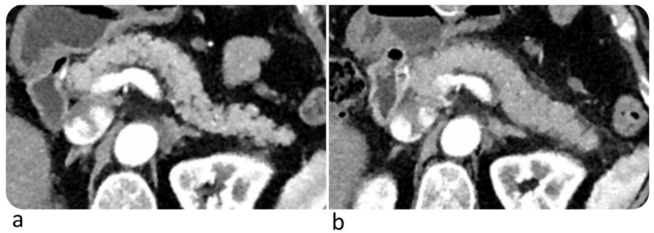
Complicated immune-related pancreatitis in a 61-year-old woman with metastatic melanoma treated with Nivolumab. CT image on arterial phase after 6° Nivolumab showed a normal pancreas (a); after 9° Nivolumab (4 months) CT showed a diffuse pancreatic enlargement associated with peripancreatic fat stranding (b). The patient was asymptomatic, but the laboratory test demonstrated an increase of lipase and amylase enzymes. © Department of Radiology, Candiolo Cancer Institute—FPO-IRCCs. Turin.
